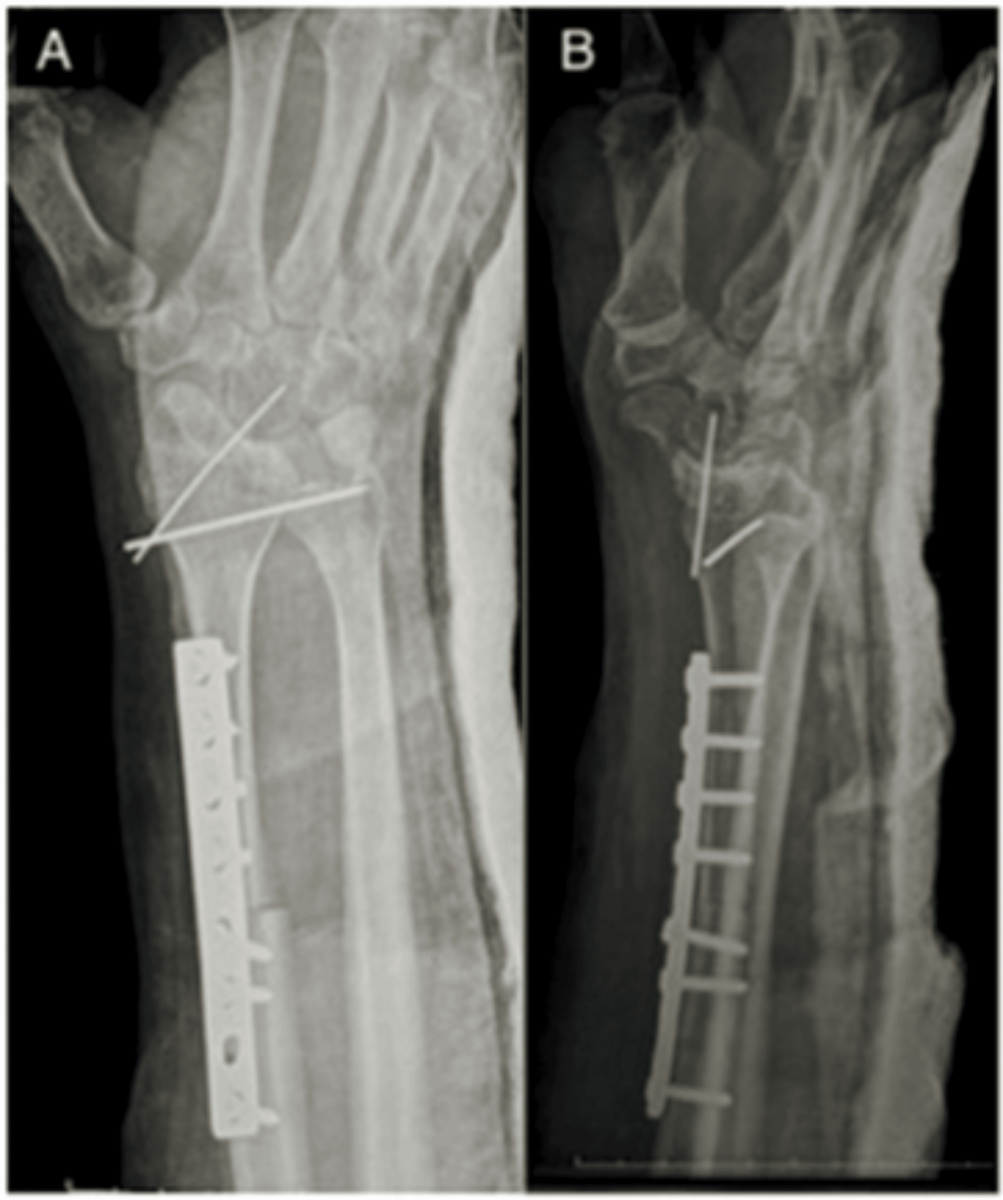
Immediate anteroposterior (A) and lateral (B) postoperative radiographs.
